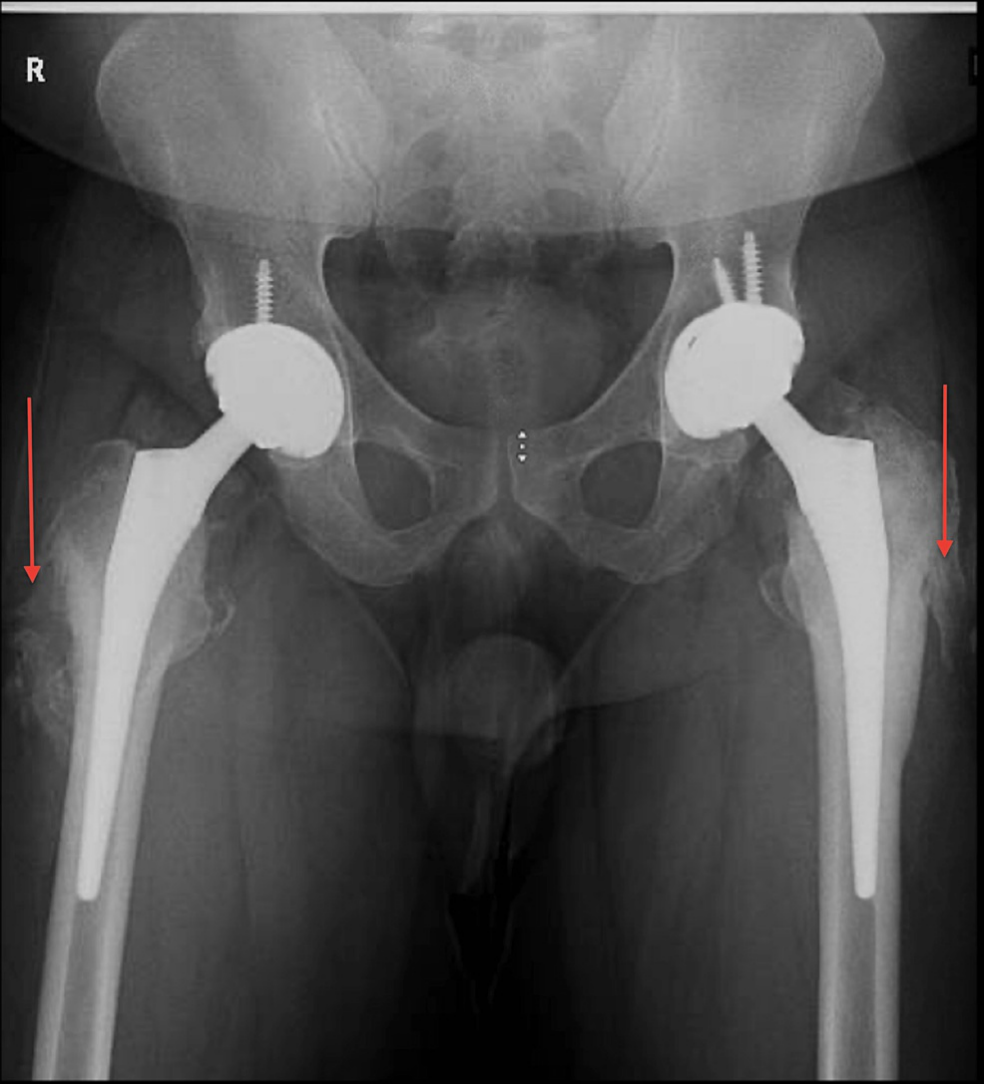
Follow-up x-ray after three years (arrows - heterotopic ossification)
